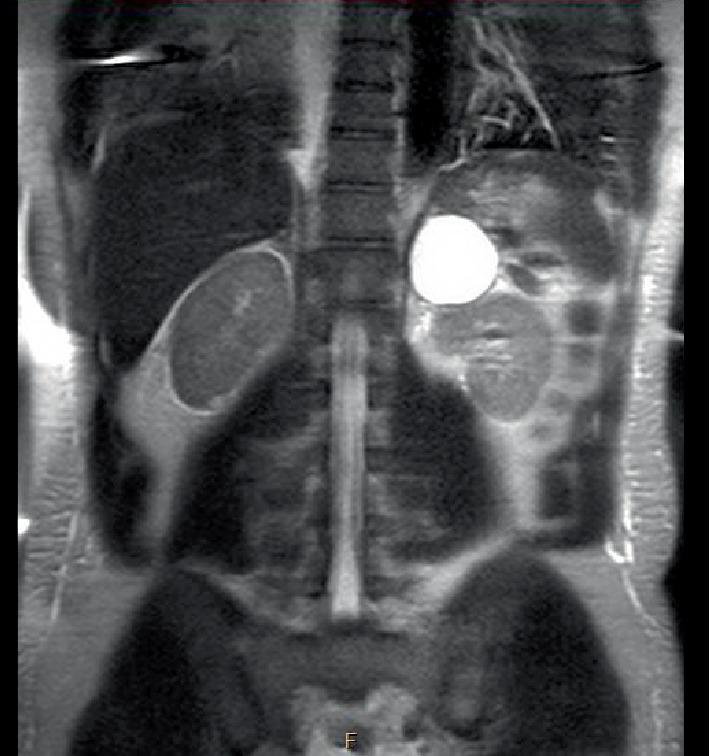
MRI abdomen demonstrating left adrenal mass.
